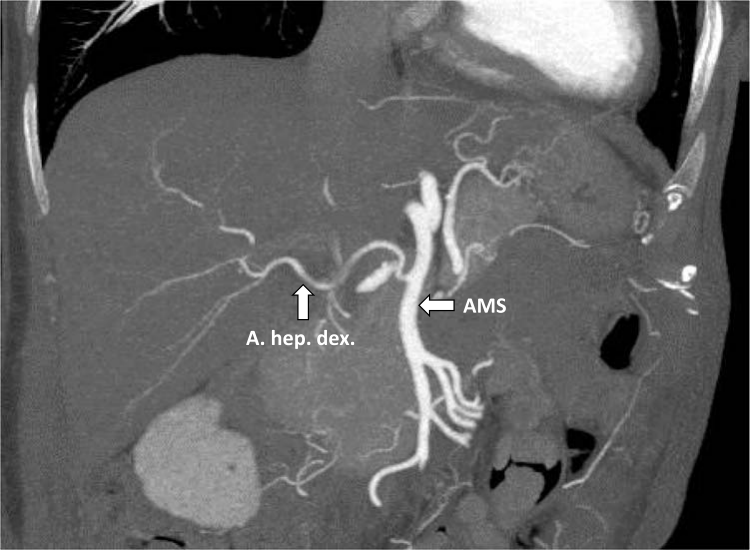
CT-angiography showing the accessory right hepatic artery originating from the superior mesenteric artery.
